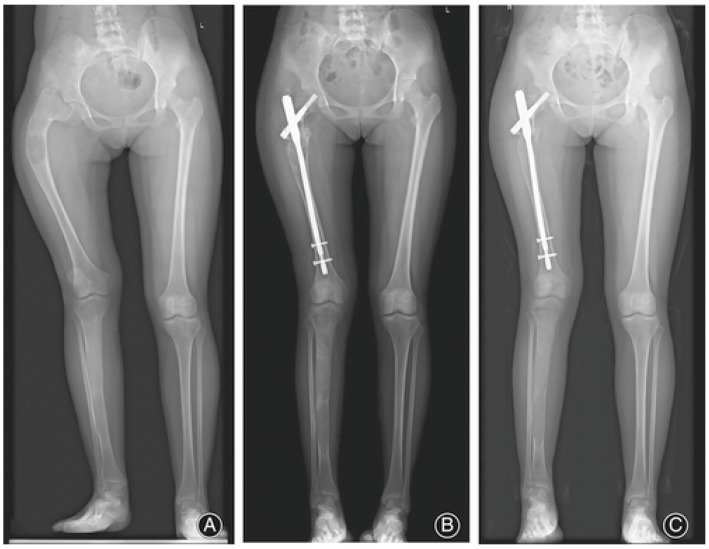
A 16-year-old patient displaying the classic coxa vara “shepherd’s crook” deformity, as well as a bone lesion in the right femur. Anterior–posterior radiographs are shown before surgical intervention (A), as well as 2 and 24 months postoperatively (B,C). Adapted with permission from Ref. [34]. 2022, *Orthopaedic Surgery*.
